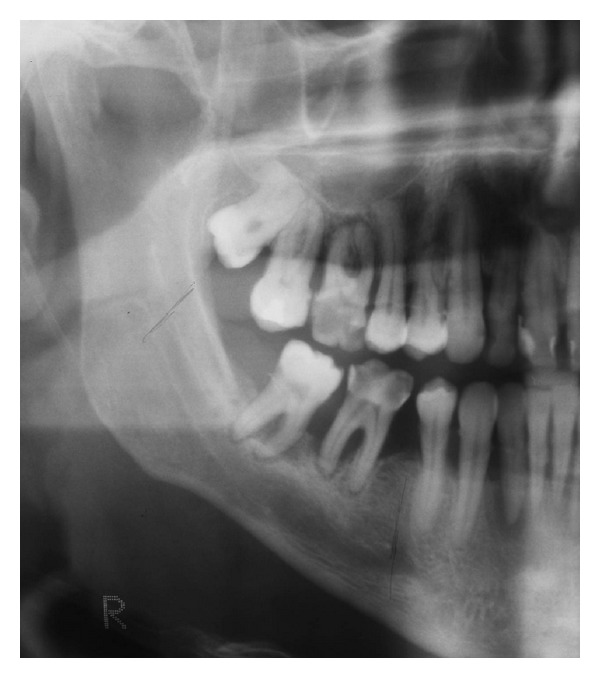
Case  3—preoperative OPTG. Beside multiple carious lesions the present X-ray examination reveals signs of chronic periodontal disease with a general loss of horizontal bone level, liberation of both dental roots, and bifurcations. The local maximum of destruction is found in the region of the last two molars. The retromolar triangle, however, appears to be intact.
